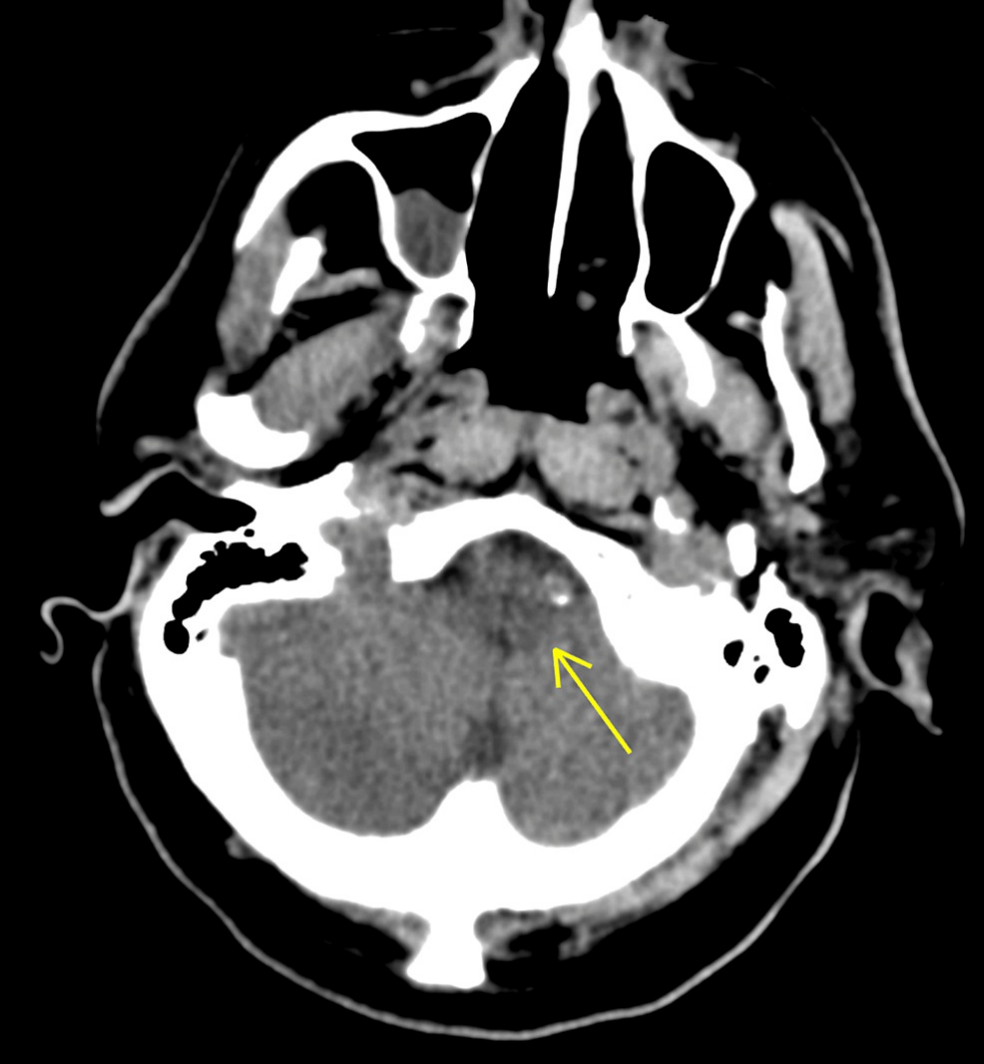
Head CT demonstrating no acute intracranial abnormality.
